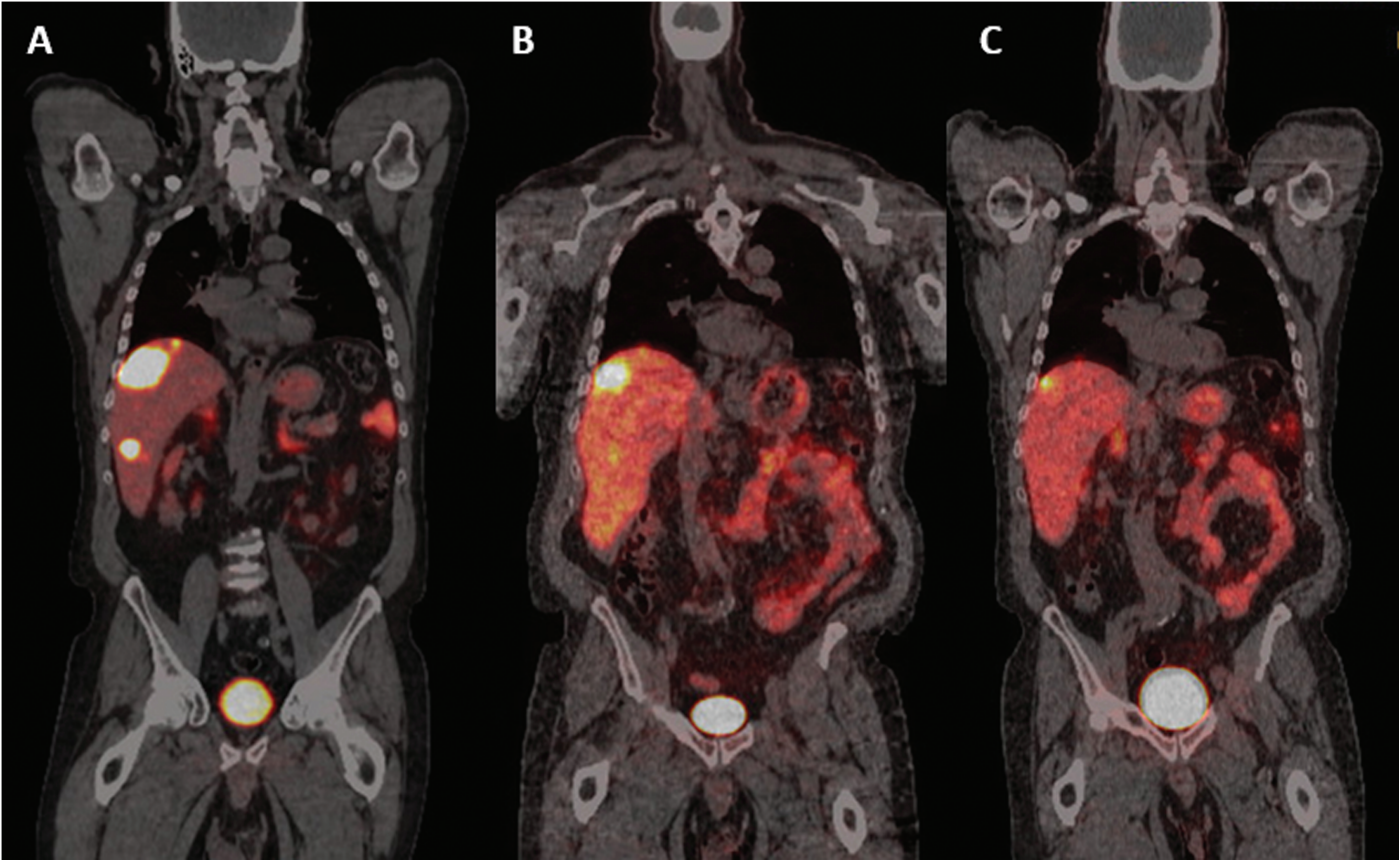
^68^Ga-Dotatate PET/CT of the patient: (A) before, (B) 1 month after, and (C) 5 months after PRRT rechallenge.
